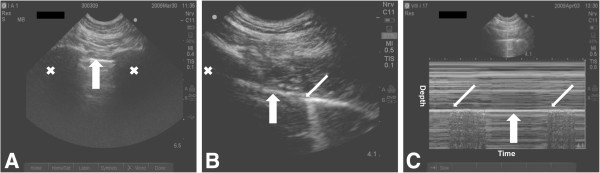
Ultrasound detection of pneumothorax. Symbols: Wide arrow, pleural line; thin arrow, lung point; X, rib shadow. A) The pleural line between two ribs is identified close to the sternum. B) The probe is rotated to align in the intercostal space and then gradually moved towards the lateral-posterior area of the chest, always following the pleural line. The aim is to detect “to and fro” horizontal movement at the pleural line, which is called lung sliding, excluding air between the pleural layers (visible only on video clips). Even with a pneumothorax present, the lung is still in contact with the interior chest wall. This contact point is called the “lung point” and moves in and out of the ultrasound screen alongside the pleural line, synchronous with respiration (visible only on video clips). The lung point is 100% pathognomonic for pneumothorax. C) In time-motion mode, the lung point appears as two distinct sonographic patterns; one pattern is suggestive of normal lung sliding (seashore pattern) and is interposed by horizontal lines (stratosphere pattern) that are seen when intrapleural air is present.
